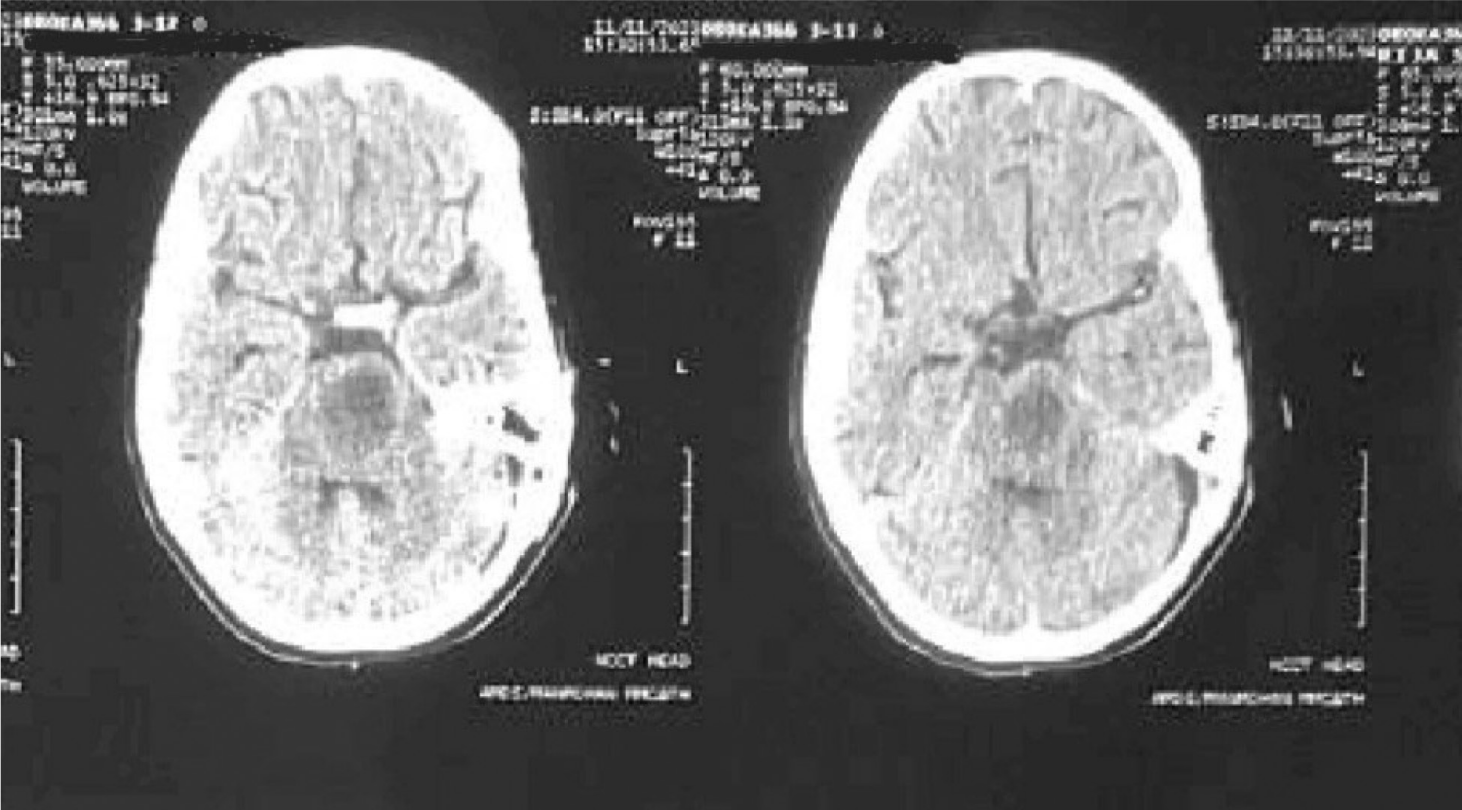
CT scan showing an ill‐defined hypo‐dense area in the left area of the brain stem predominantly pons.
